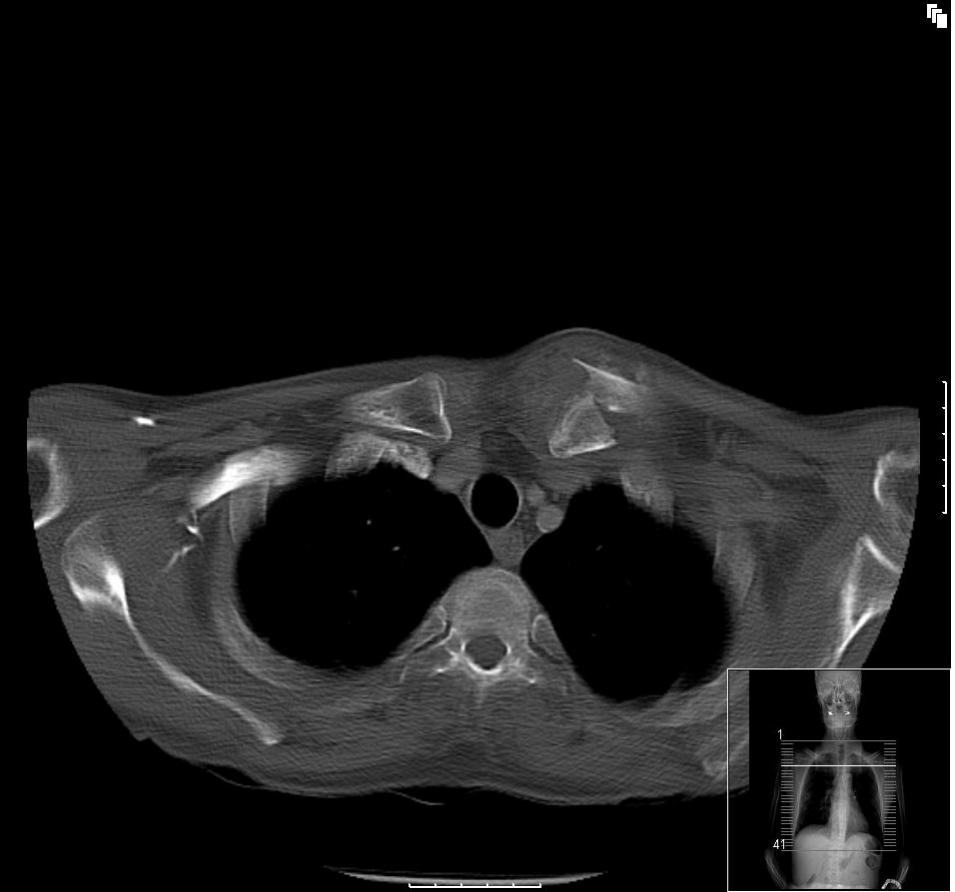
Axial CT image showing a displaced fracture of the left clavicle (day 8).
